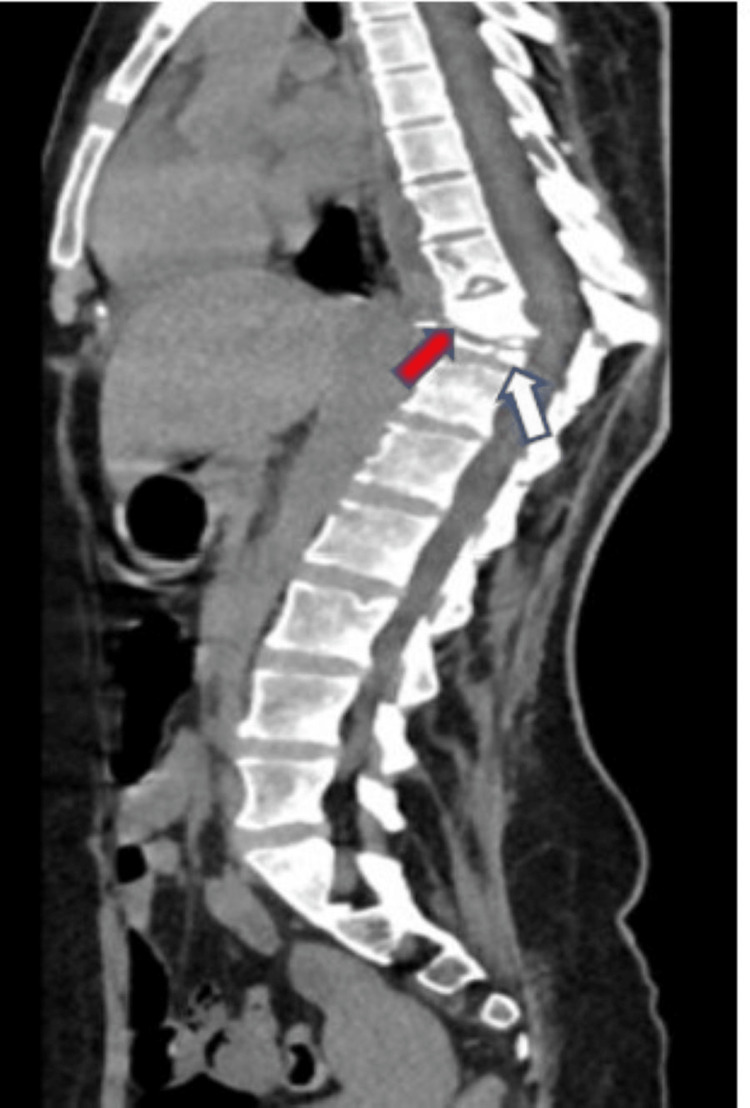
Sagittal non contrast CT of the dorso-lumbar spine reveals lytic destruction complete collapse of the D11 vertebra (white arrow) and partial antero-inferior wedge collapse of the D10 vertebra (red arrow) with bony fragments in the prevertebral soft tissue
